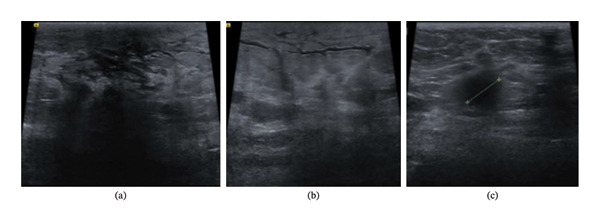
(a, b and c): A 43‐year‐old patient with diagnosis of left breast carcinoma presented with left mastodynia for 2 months. The ultrasound of the affected left breast shows diffuse subcutaneous oedema with thickening of the skin layer (a and b). In c, a hypoechoic, globular, ipsilateral axillary adenopathy is shown, without recognizability of the adipose hilum.
